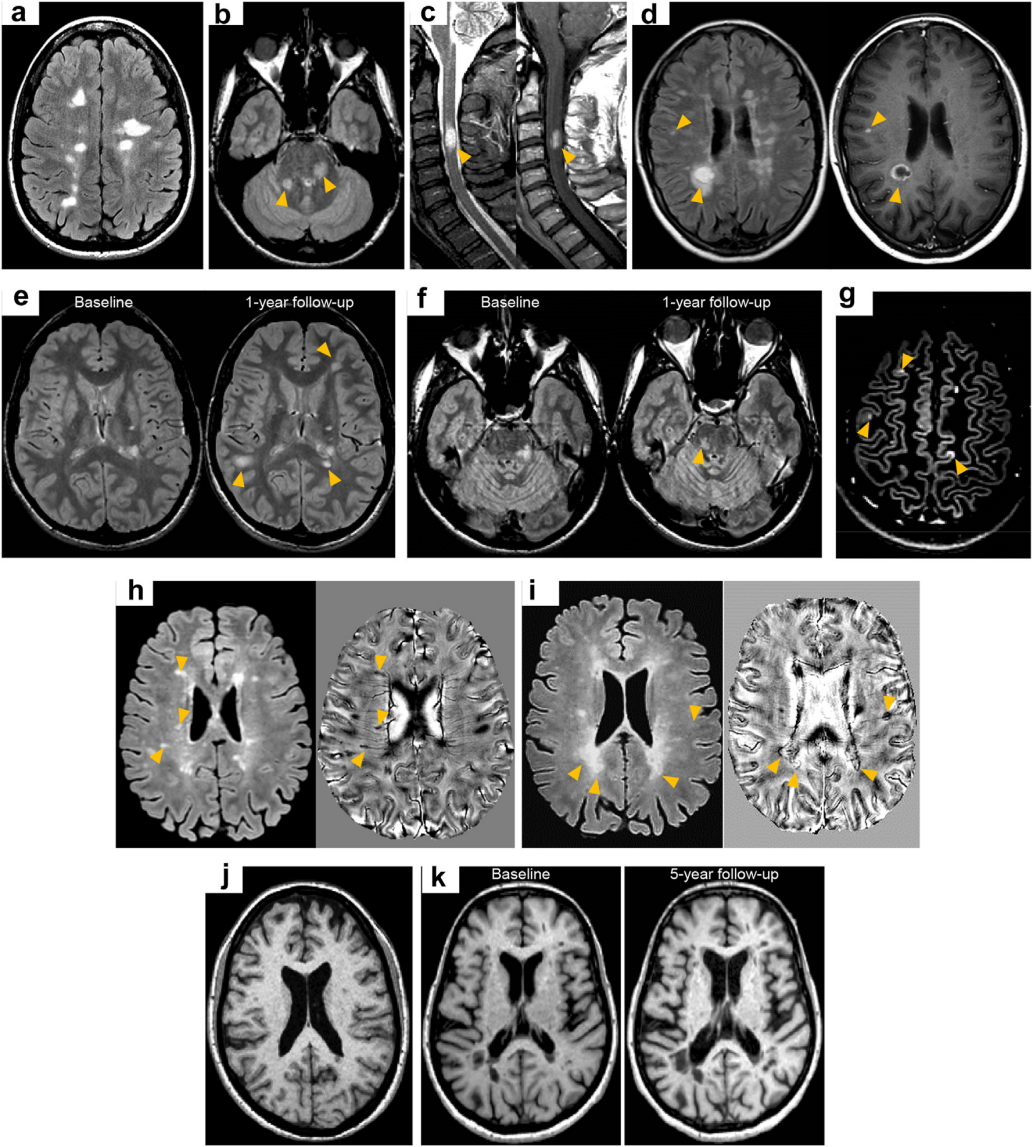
Summary of early MRI predictors of subsequent worse disease disability progression and evolution to secondary progressive multiple sclerosis at disease onset. (a) The number and volume of brain T_2_-hyperintense white matter lesions; (b) ≥1 infratentorial lesion (orange arrowheads); (c) ≥1 spinal cord lesion at baseline (orange arrowheads); (d) ≥1 gadolinium-enhancing lesions at baseline (orange arrowheads); (e) increase of T_2_-hyperintense brain white matter lesion number and volume (especially of deep white matter) during the first 1–5 years; (f) ≥1 infratentorial T_2_-hyperintense white matter lesions within 1–3 years (orange arrowheads); (g) ≥1 cortical lesion at baseline (orange arrowheads) on double inversion recovery sequence. Further promising early MRI markers are h) the presence of lesions with the “central vein sign” on susceptibility-based MRI (orange arrowheads); (i) the presence of paramagnetic rim lesions on susceptibility-based MRI (orange arrowheads) (j) presence of substantial brain volume loss at disease onset and/or (k) a faster rate of brain atrophy in the first years of the disease.
